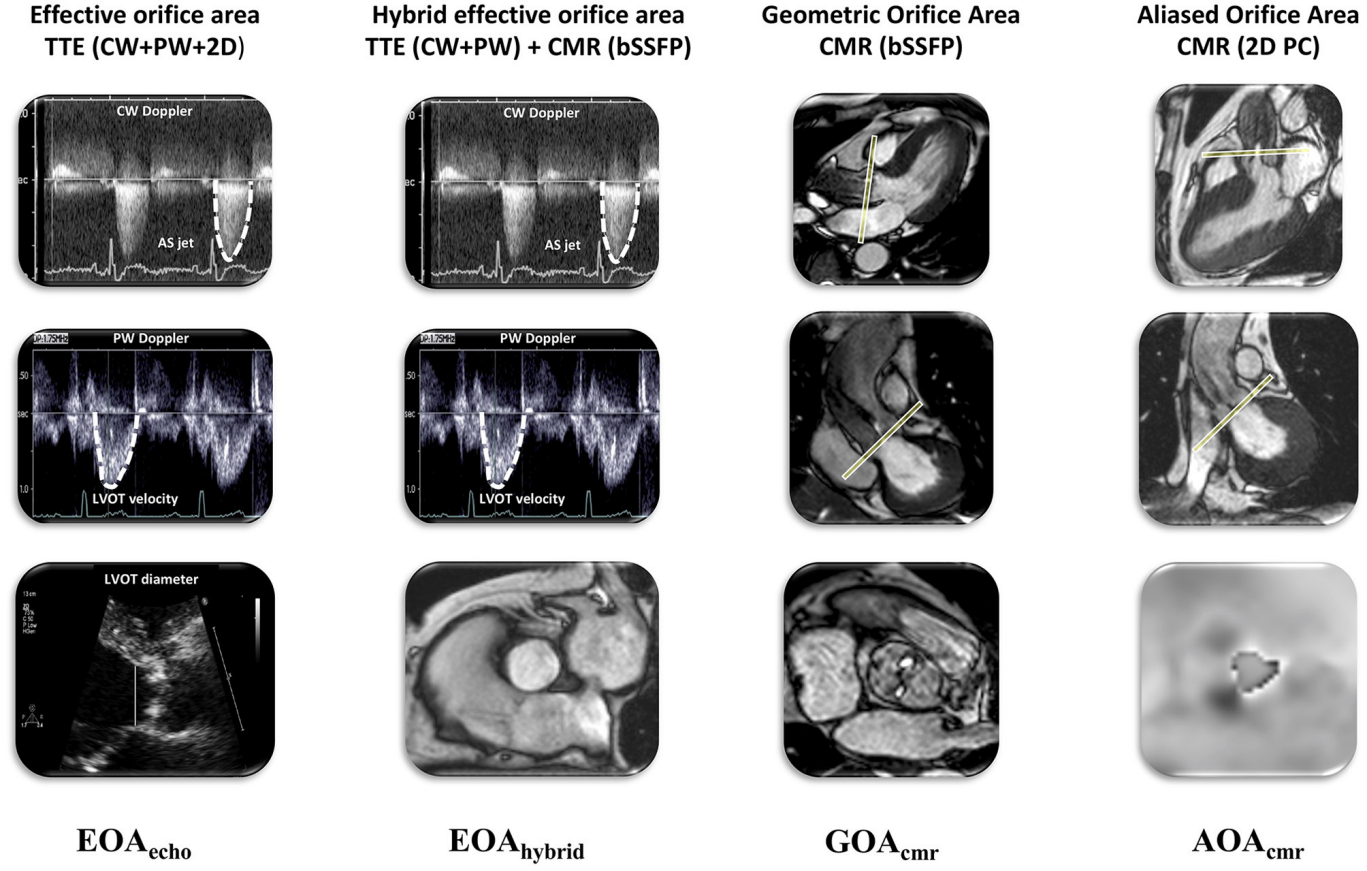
Summary of different methods for non-invasive assessment of aortic valve area and grading of aortic stenosis severity: EOA by TTE, hybrid EOA by TTE and CMR, GOA and EOA by CMR. AOA, aliased orifice area; EOA, effective orifice area; CMR, cardiovascular magnetic resonance; GOA, geometric orifice area; echo, echocardiography; hybrid, combined echo-CMR assessment; TTE, transthoracic echocardiography.
